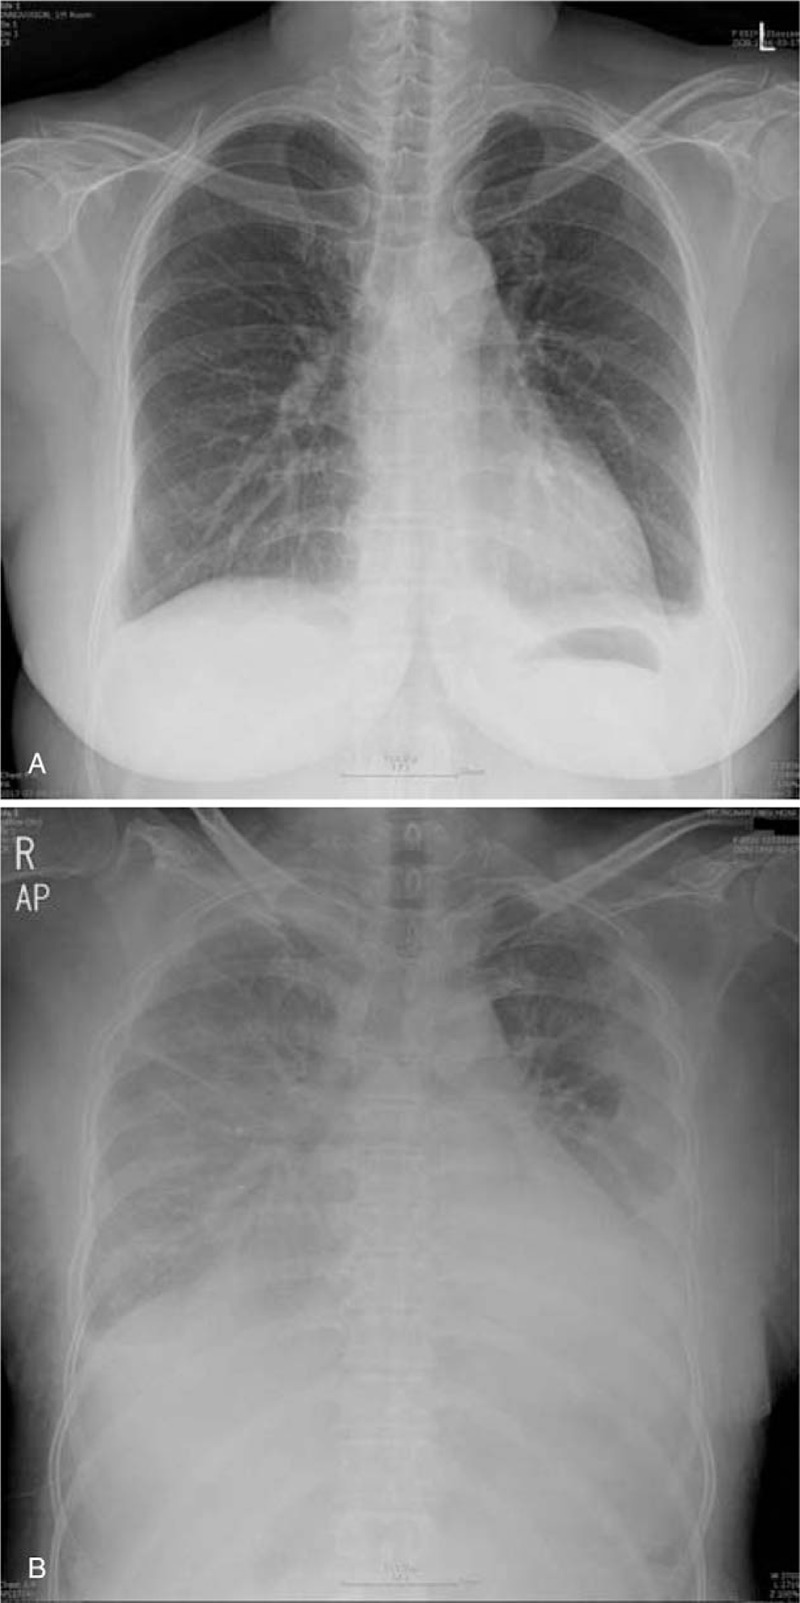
Chest X-ray. (A) On admission, bilateral pleural effusion was detected. (B) Three days later, pulmonary edema developed and pleural effusion was aggravated.
